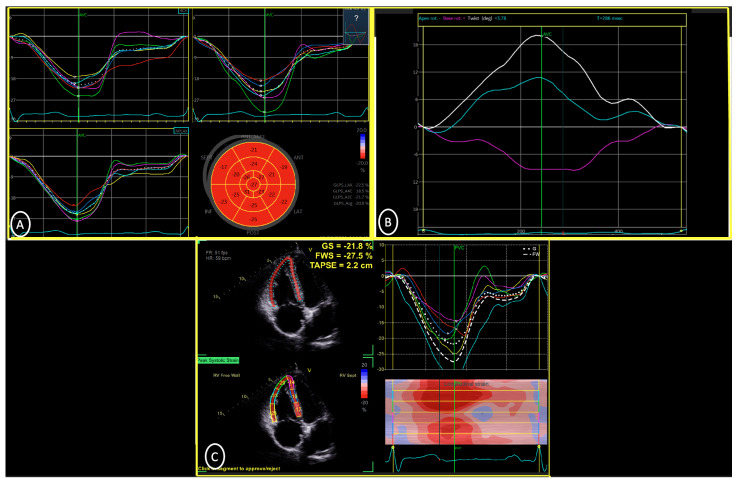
Cardiac mechanics assessed in the FIT-TWIST study. Examples of the cardiac mechanics assessments performed as part of the FIT-TWIST study. (A) LV GLS assessment depicted in segmental curves, a bullseye plot, and values. (B) LV twist and torsion, with apical (light blue), basal (purple), and net twist (white) depicted. (C) RV GLS and RVWS depicted in segmental curves, color M-mode, and values. 2CH = apical 2-chamber; 4CH = apical 4-chamber; AVC = aortic valve closure; AVG = average; GLS = global longitudinal strain; GLPS = global longitudinal peak strain; GS = global strain; LAX = apical long-axis; LV = left ventricle; ROT = rotation; RVFWS = right ventricular free wall strain; TAPSE = tricuspid annular plane systolic excursion.
